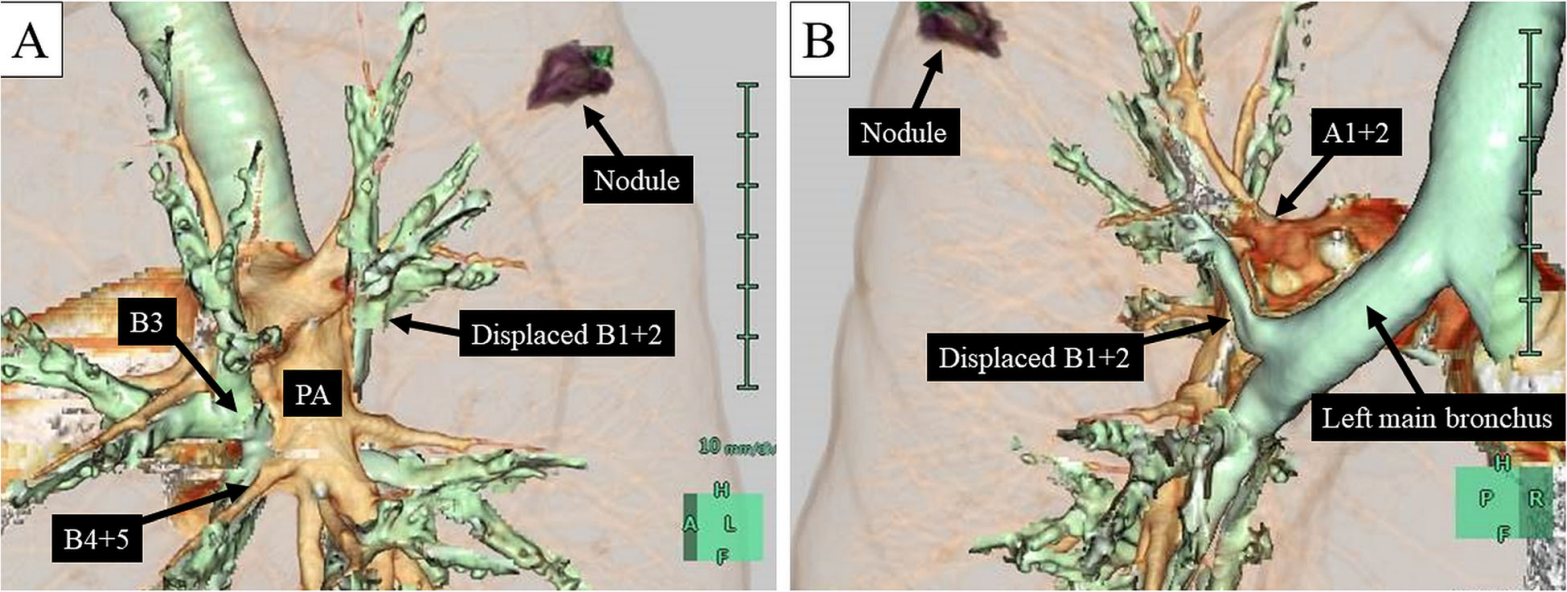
Preoperative three-dimensional chest computed tomography images. a Preoperative three-dimensional computed tomography reconstruction imaging revealed a displaced apicoposterior bronchus (B^1 + 2^) branching behind the main pulmonary artery. The nodule was located in the left apicoposterior segment (A^1 + 2^). b A branch of the pulmonary artery of the left A^1 + 2^ branched from the main pulmonary artery along the head side of the displaced B^1 + 2^. PA: pulmonary artery
